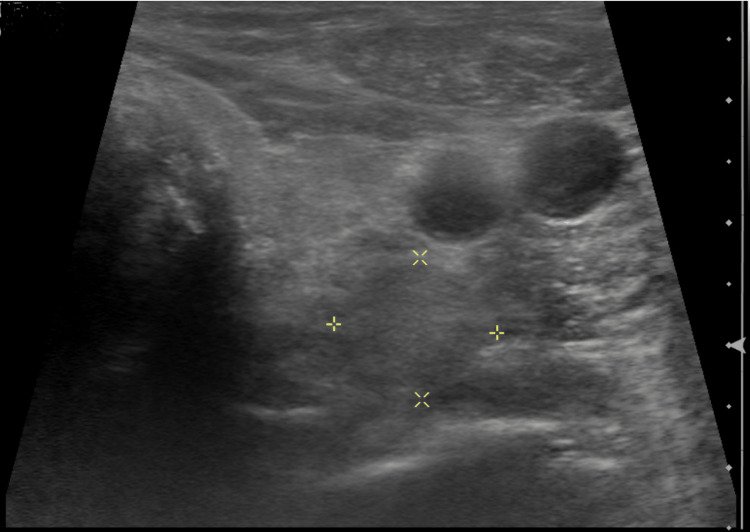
An ultrasound of the neck shows a solid area located lateral and inferior to the left thyroid gland.
